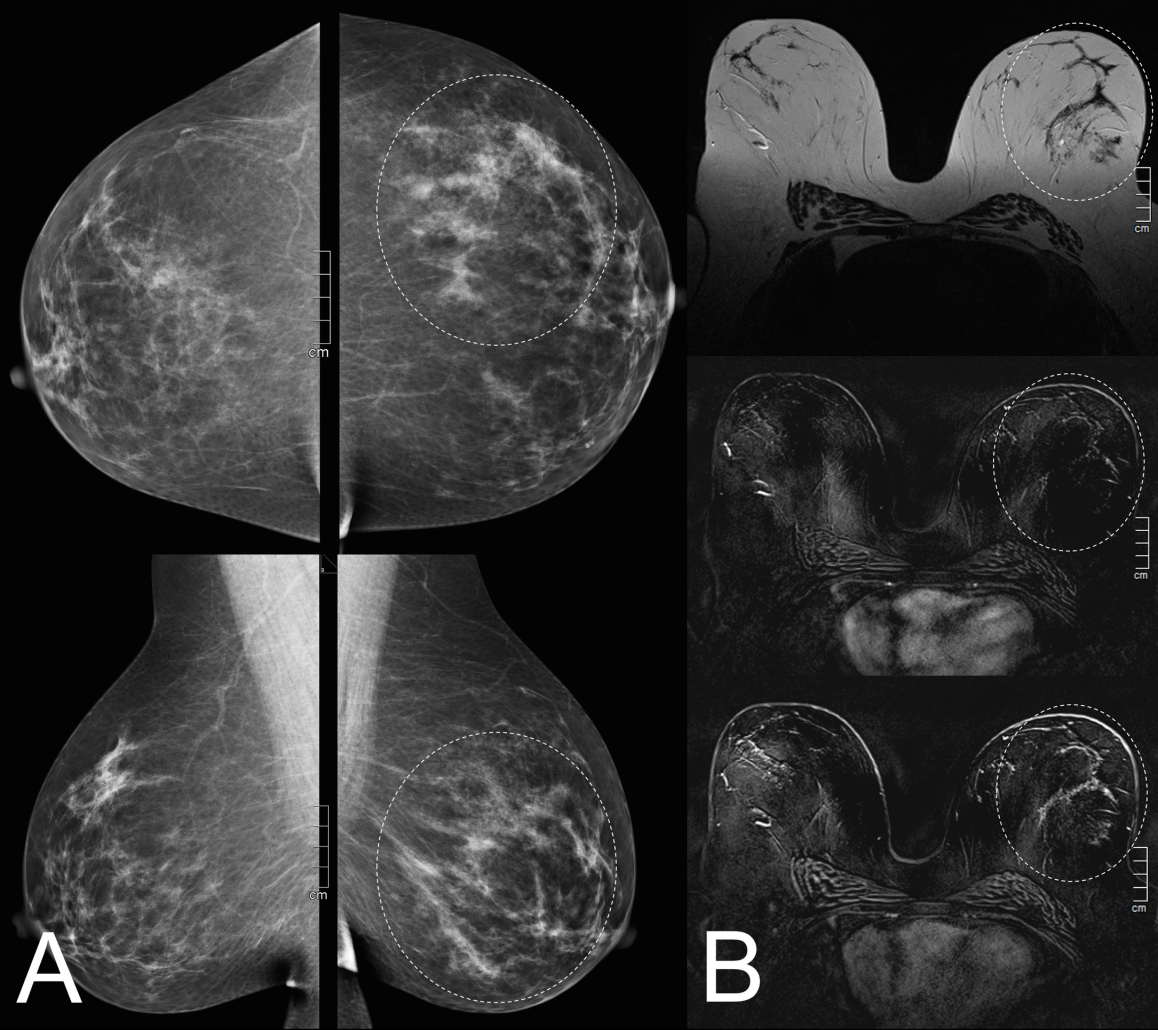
A 52-year-old patient referred for problem-solving due to newly diagnosed architectural distortion in the left breast (A; white circles on mammography images). 3T contrast-enhanced MR imaging (B; top: T2w image, middle: early contrast-enhanced image, bottom: late contrast-enhanced image) shows the architectural distortion (white circle) demonstrating only mild background enhancement. The lesion was classified as BI-RADS 2, definitely benign. Follow-up of two years did not reveal malignancy.
